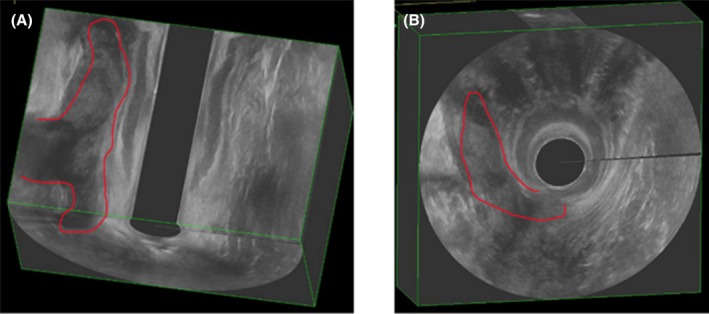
Endoanal ultrasound of a high transphincteric fistula with a large secondary tract. Three‐dimensional endoanal ultrasound. (A) Coronal plane showing the secondary tract along the anal canal (red contour). (B) Axial (transverse) slice of the anal canal with the corresponding tract outlined in red. The central artefact corresponds to the probe footprint.
